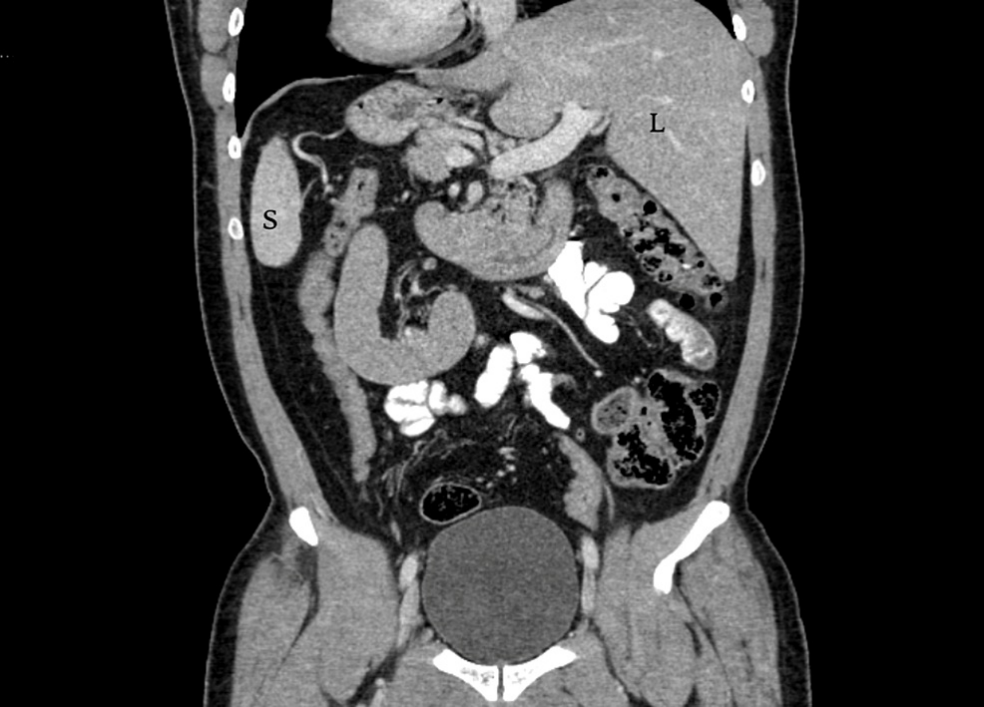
A Coronal view of an abdominopelvic CT scan showing a reversed location of the abdominal organs with the liver (L) positioned in the left side and the spleen (S) being in the right side
